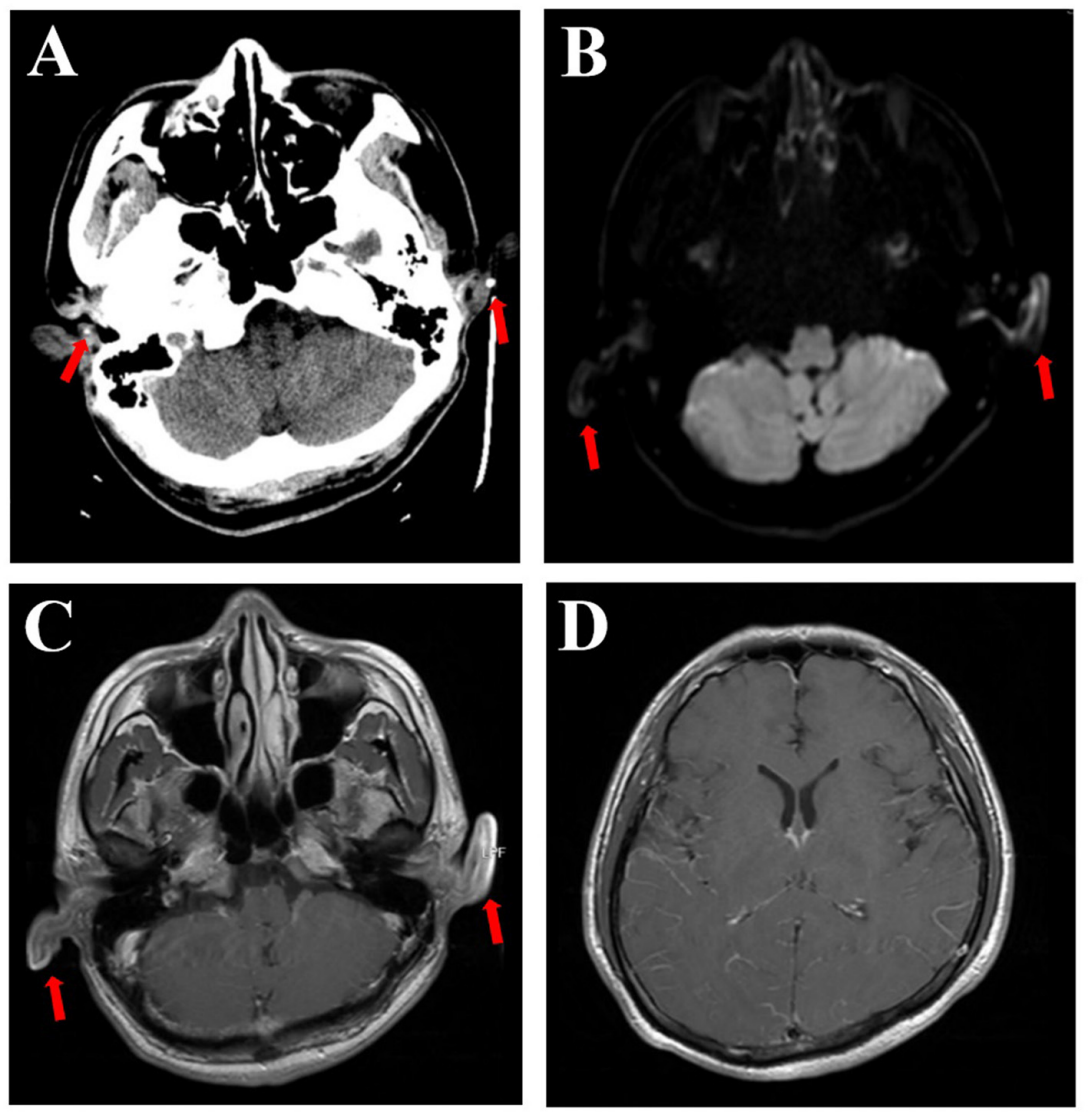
Brain CT scan showing calcification (arrows), which can be seen in the polychondritic ears (A). DWI shows abnormal signals in the bilateral auricles (B, arrows). T1-weighted MRI with contrast showed abnormal signals and thickening of both ears (C, arrows) and increased enhancement of the pia mater (D).
